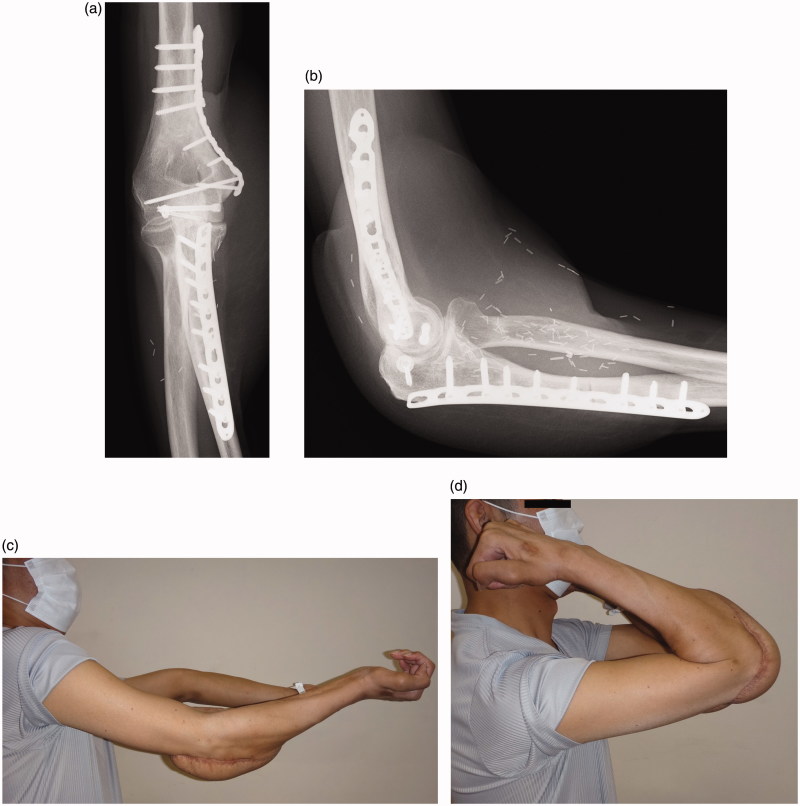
Follow-up radiograph and photograph obtained 2 years after the surgery. Anterior-posterior (a) and lateral (b) radiographs showing bony union of the osteotomy site, with no evidence of osteoarthritic changes at the elbow joint. Clinical photograph showing functional results in extension (c) and flexion (d).
